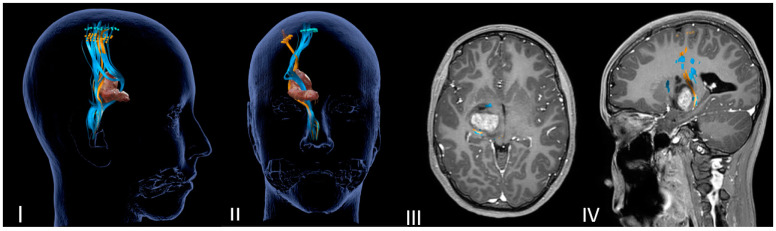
Neuronavigated transcranial magnetic stimulation pre-op study. (I,II) A 3D reconstruction of the nTMS data that shows cortical maps of the right spinal tract (CST) for the foot (blue) and hand (orange) and its relationship with the thalamopeduncular tumor of Case A. (III,IV) Brain MRI merged with nTMS data showing that the cortical spinal tract (CST) runs antero-medially respect to a right thalamopeduncular tumor.
